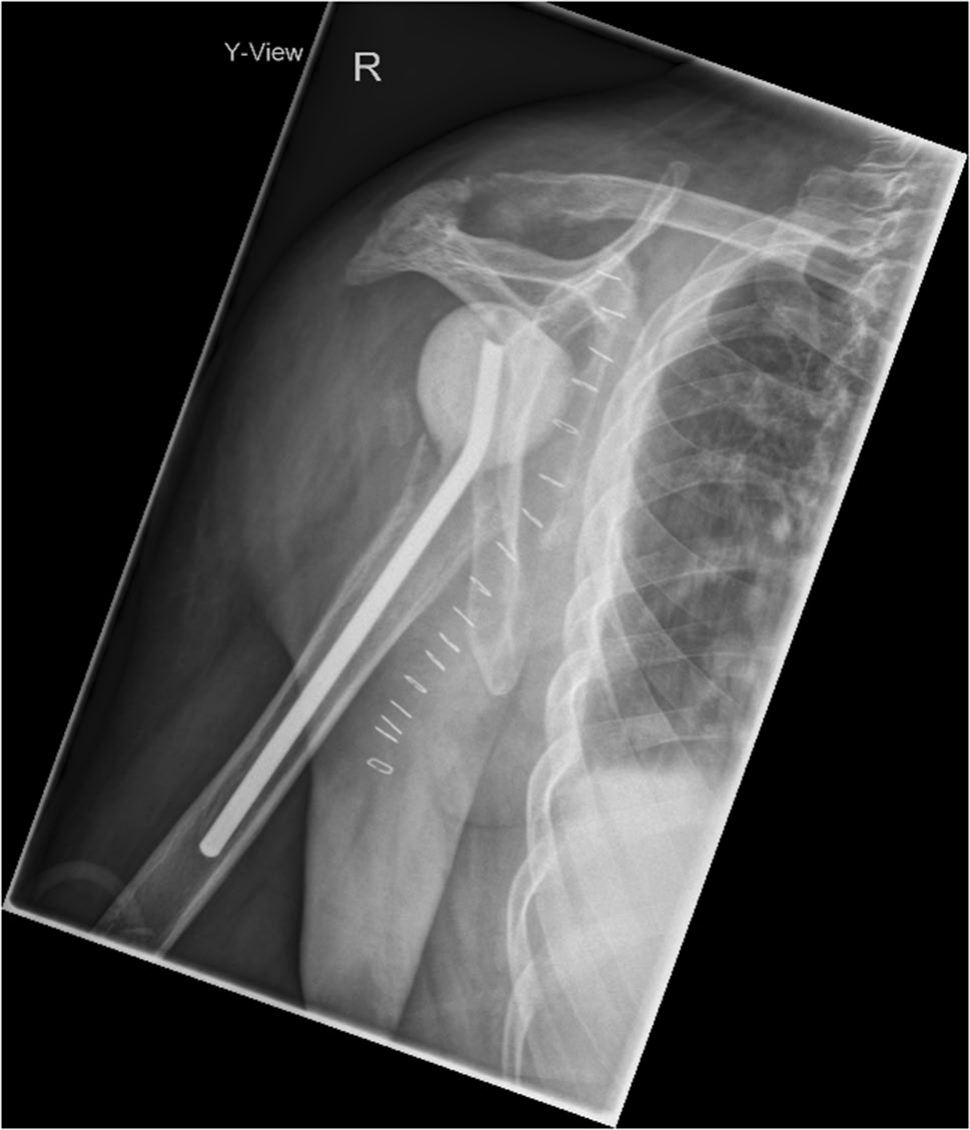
Radiographic examination of the right shoulder joint (*Y*-view), following the explantation of the hemi prosthesis and implantation of an antibiotic-impregnated bone cement spacer
